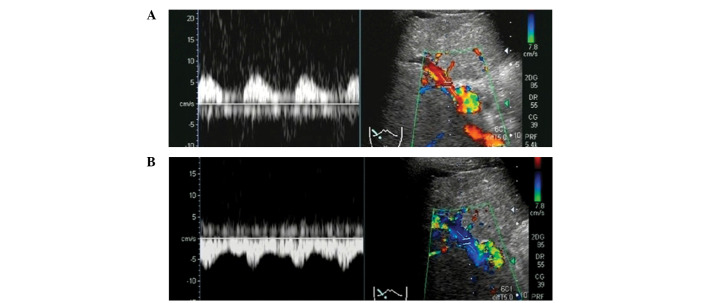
Abdominal ultrasound images. (A) Fast Fourier Transform (left) and color Doppler ultrasongraphy (US) (right) images during deep expiration reveal hepatopetal blood flow (red) in the right portal vein branch. (B) Fast Fourier Transform (left) and color Doppler US (right) images during deep inspiration demonstrate hepatofugal blood flow (blue). Images were acquired in February 2012.
